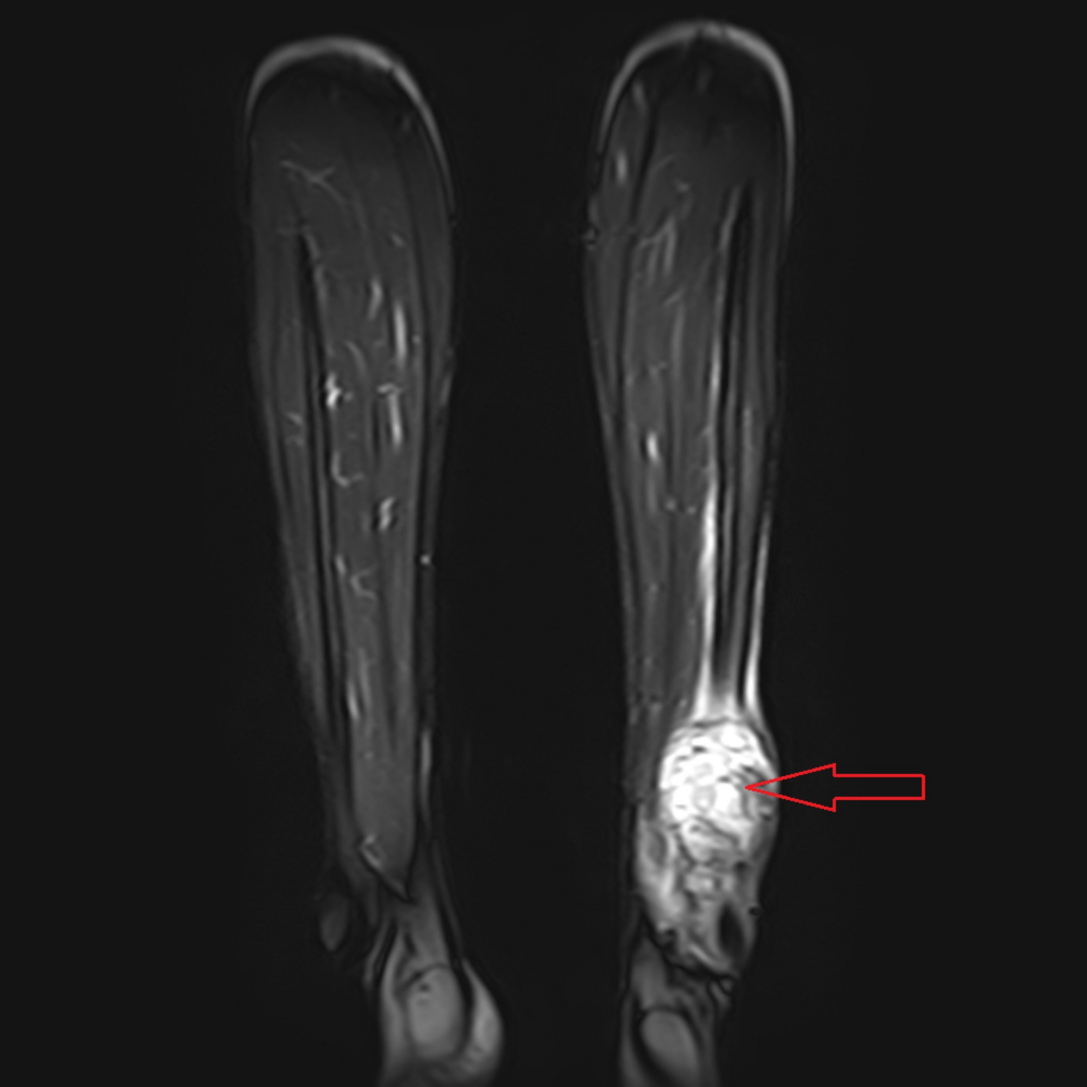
Contrast-enhanced magnetic resonance imaging (MRI) scan of the lower extremities. Red arrow shows a well-defined multicystic mass arising from the distal metaphyseal region of the left fibula.
